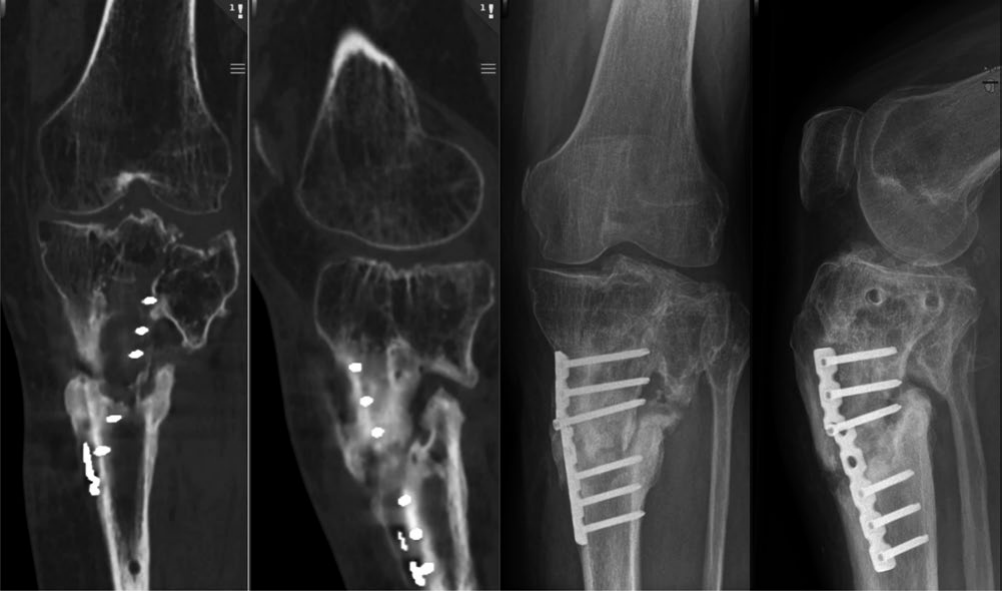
Preoperative CT and radiographs showing posttraumatic lateral tibial joint incongruence and non-union.
